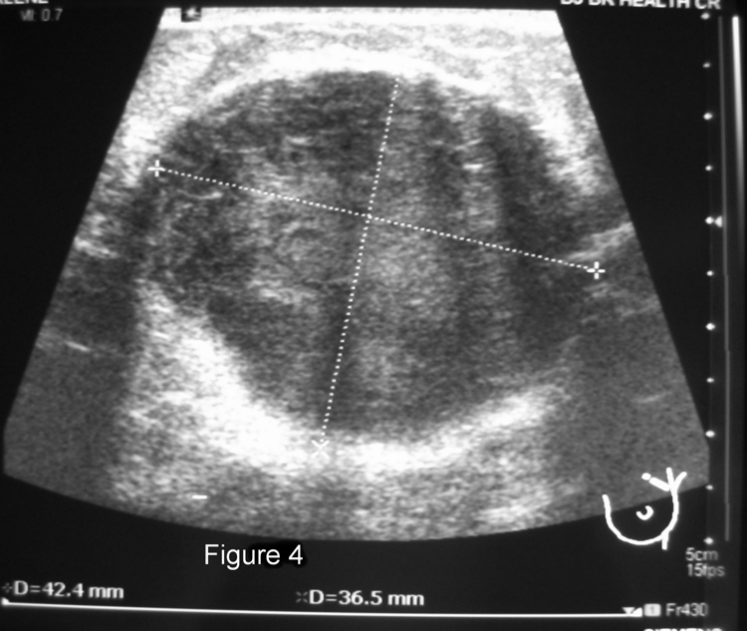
Left breast ultrasound from a 35 year-old patient with bilateral palpable breast masses. The ultrasound documented a 42 × 36 mm hypoechoic mass. She was found to have metastatic disease to her breast from a lung neuroendocrine carcinoma.
